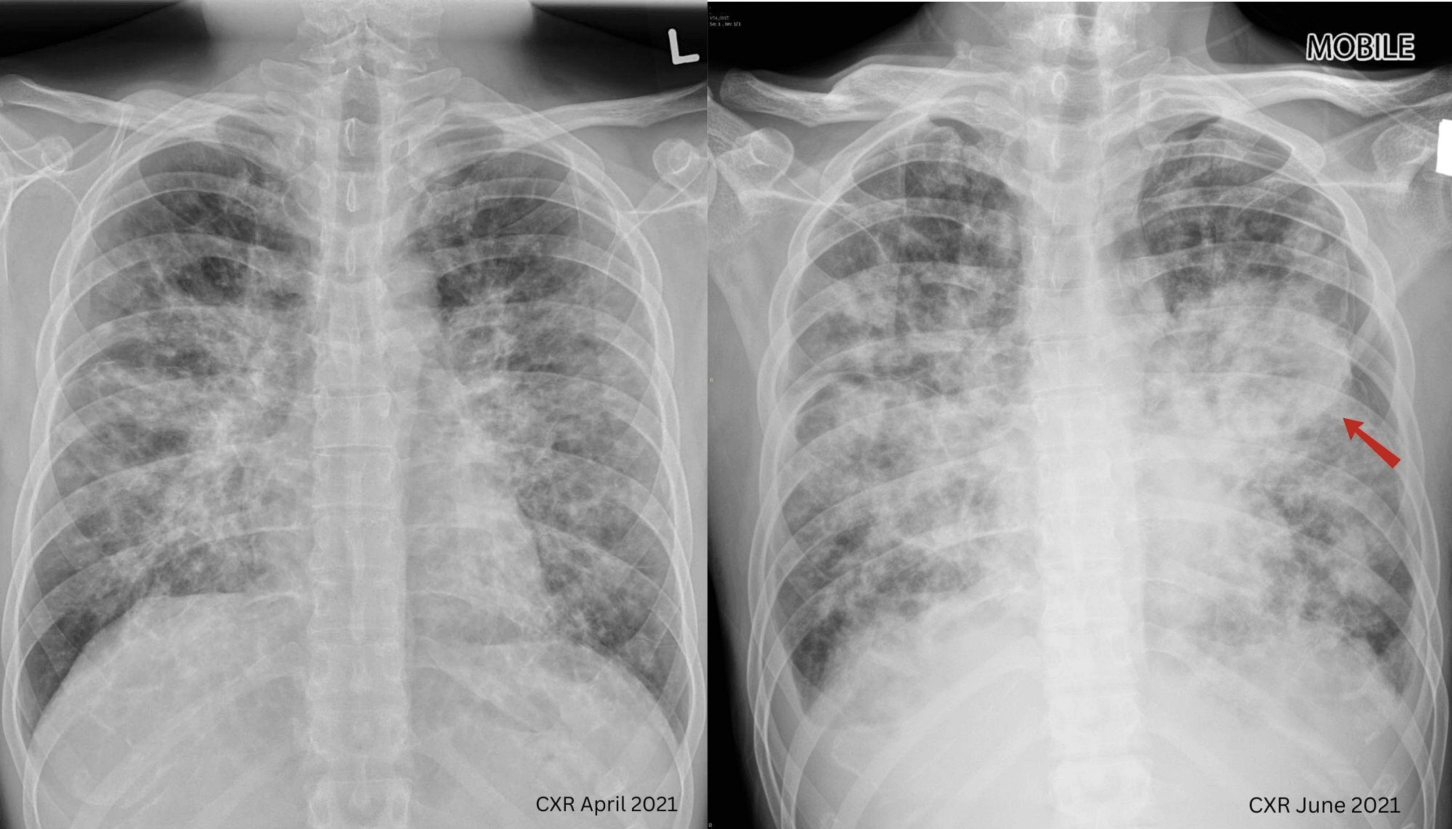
A comparison of the CXRs of the patient: first admission in April 2021 vs. June 2021 It demonstrates the progression of bilateral infiltrates from interstitial and nodular appearances to a mass-like infiltrate over the left side (red arrow). There was no radiological improvement from admission despite treatment with antibiotics, anti-TB, and treatment for PCP CXR: chest X-ray; PCP: pneumocystis pneumonia
